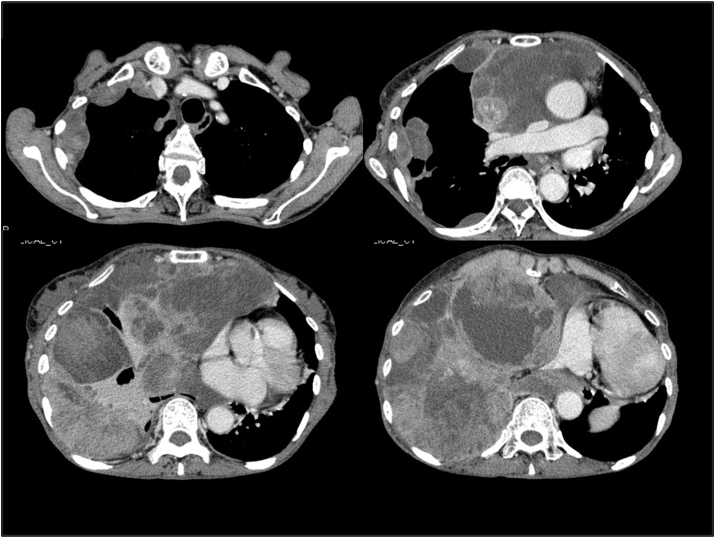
Contrast-enhanced CT scans of the chest (May 2010) The right thoracic cavity is filled with multiple masses of various sizes, and part of the masses spread to, and compressed, the left mediastinum. The interior of more than half these masses was extremely hypodense.
